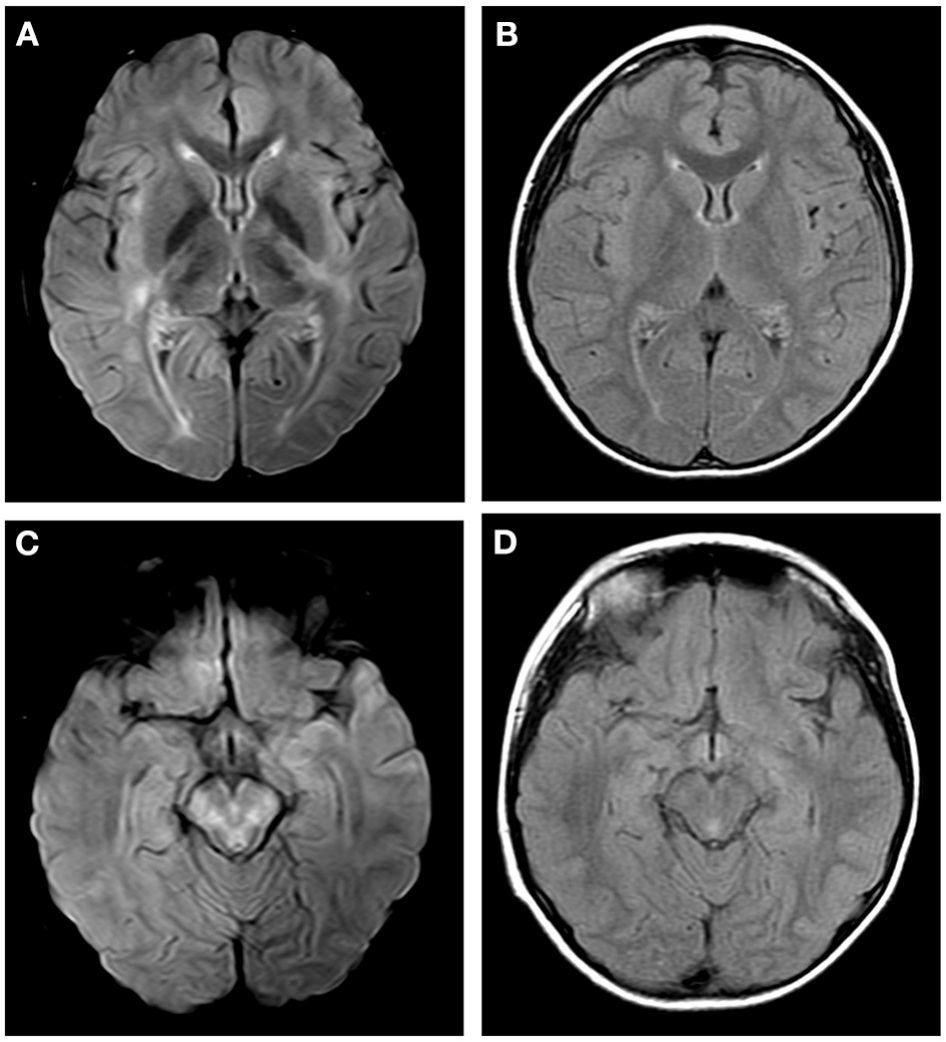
Axial fluid attenuation inversion recovery (FLAIR) MRI brain (A,C) 12 days after admission and (B,D) 1 month later. Image (A) showed T2-hyperintense lesions in internal capsule, hind limbs and external capsule. Image (C) showed T2-hyperintense lesions in brain stem. Follow-up images (B,D) showed improved T2 lesions.
